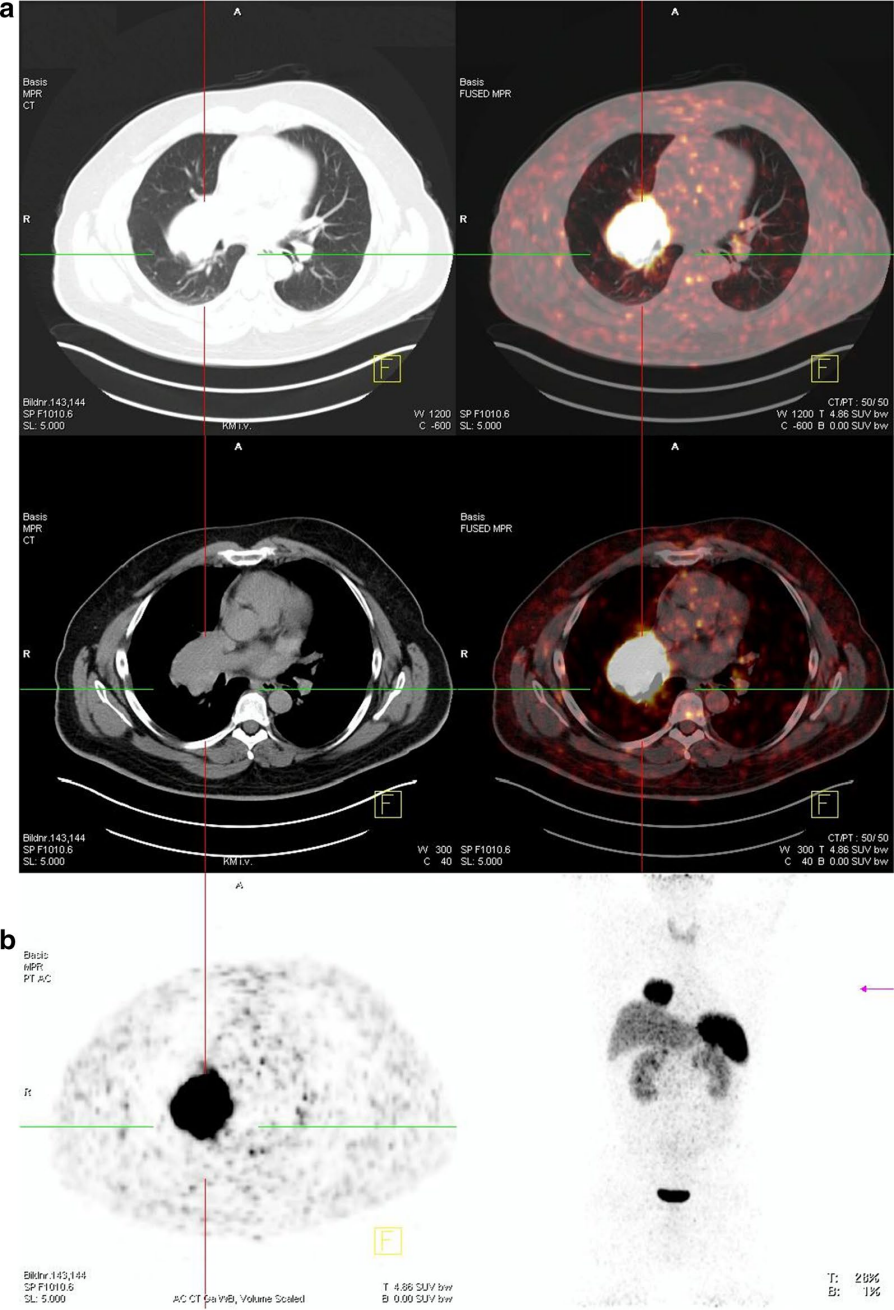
^68^Gallium-DOTATOC PET-CT before surgery revealed a large tumor mass located in the *right* lung with high somatostatin receptor expression. Isolated CT- and PET component (a
